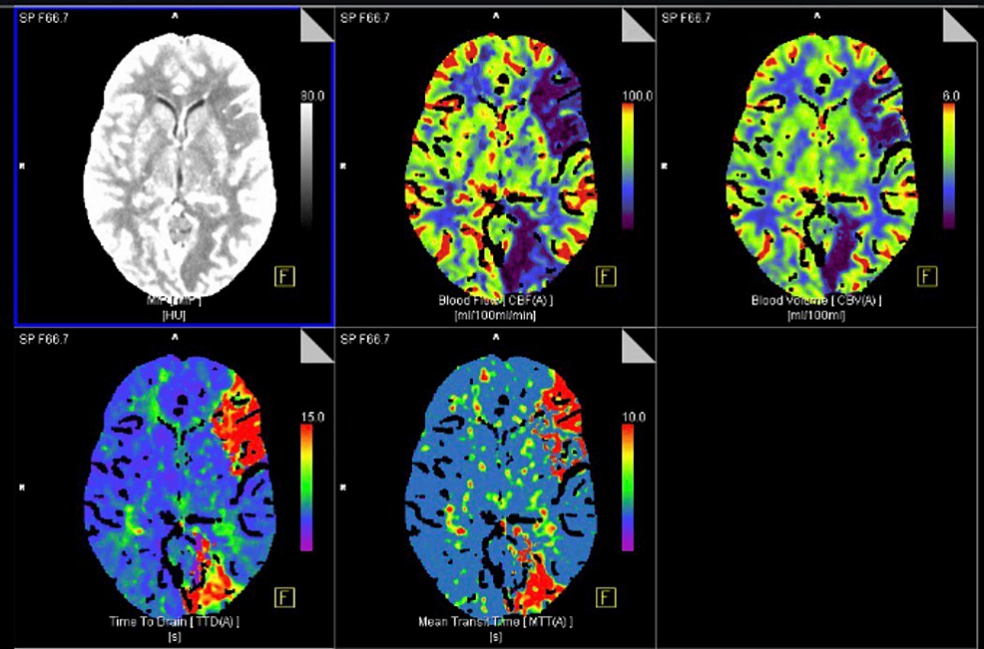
Computed tomography perfusion shows ischemic insult leading to hypo perfusion of the left frontal and occipital regions.
